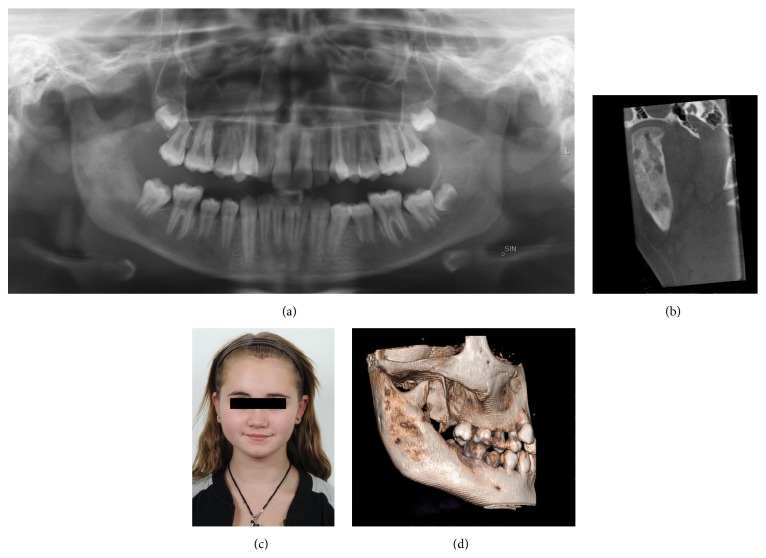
Six-month postoperative control radiographs, CBCT, and clinical image of patient case 2 with PCO. (a) Panoramic radiograph; (b) CBCT. Coronal section of the sclerotic collum mandibulae with lytic lesions; (c) patient photo, with reduced facial swelling at the right side; and (d) CT scan 3D model with bone thickness at the right side of the collum mandibulae.
